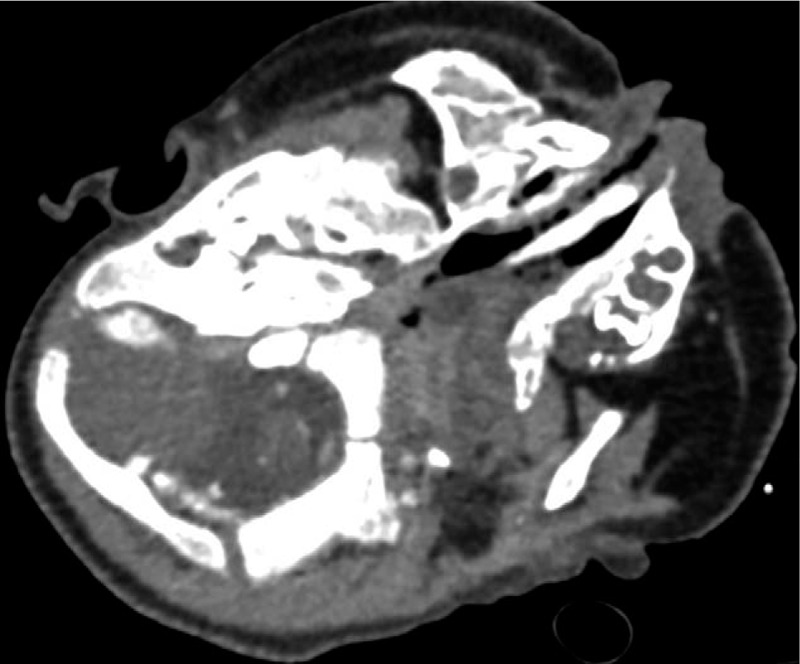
Nasopharyngeal contrast-enhanced computed tomography shows a soft tissue density mass in the left nasopharyngeal cavity and the posterior wall of the oropharyngeal cavity. The lesion is 3 × 1.9 × 1.9 cm, and is unevenly enhanced with an unclear boundary between the lesion and nasopharyngeal adipose tissue.
